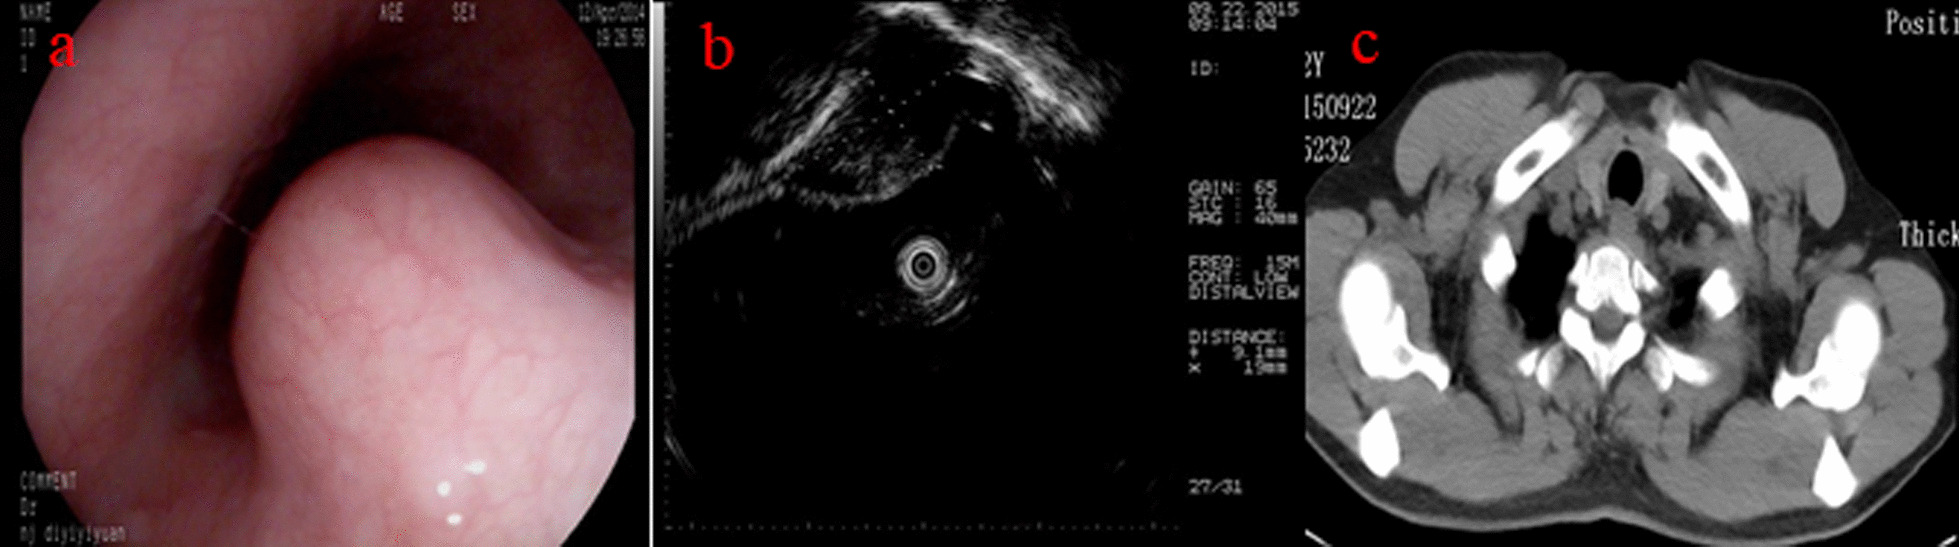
a Endoscopic image showing a 20 × 20 mm bulging lesion in the proximal esophagus. b Endoscopic ultrasound revealed a hypoechoic cystic lesion arising from the muscularis propria. c Computed tomography revealed an obvious mass located in the proximal esophagus
